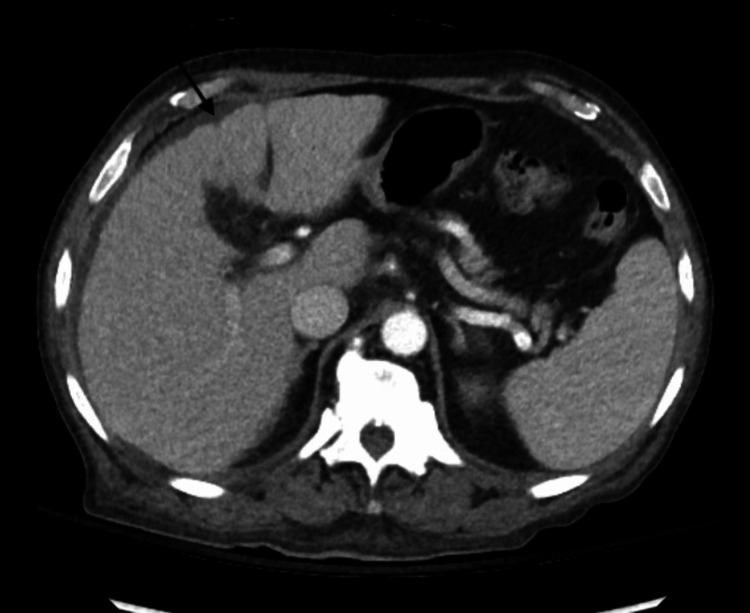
CT scan abdomen and pelvis with contrast Subtle nodular contour of the liver suggestive of cirrhosis
